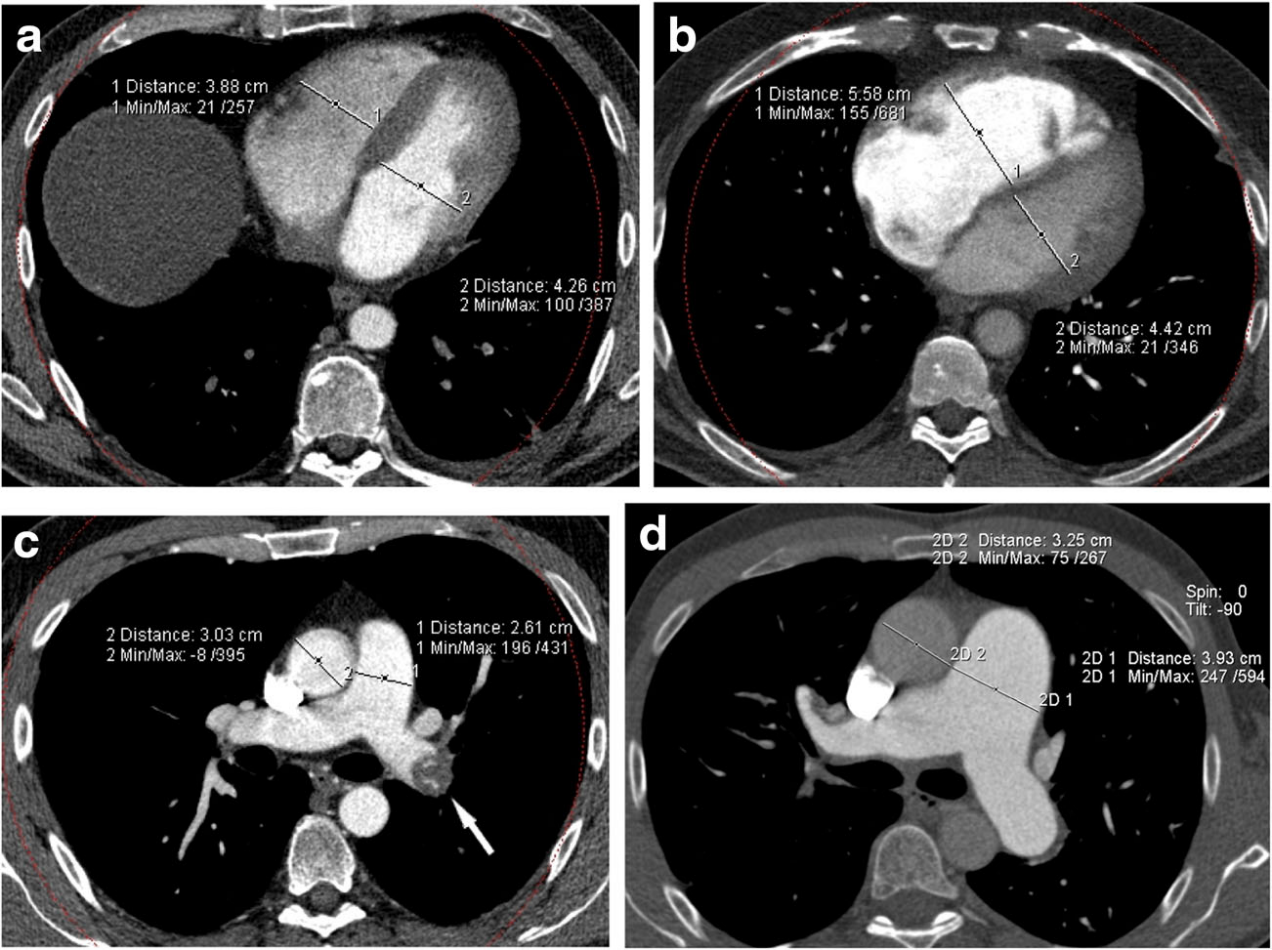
CT signs of pulmonary hypertension. Normal heart diameters in CTEPD (a); in CTEPH patients (b) right ventricle dilation with septum bowing and heart leftward rotation are present. Important main pulmonary artery dilation in CTEPH (d), absent in CTEPD (c); proximal left main artery obstruction is evident in (c) (white arrow)
